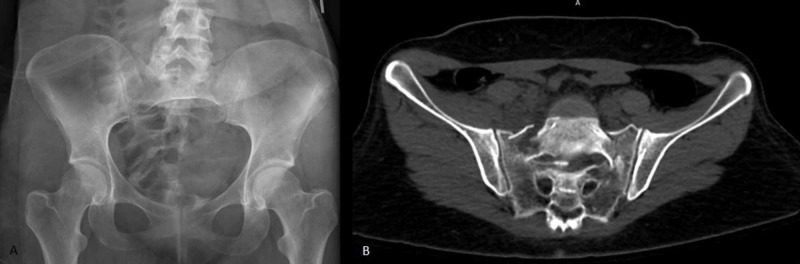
U-type sacral fracture injury films. (A) An AP pelvic radiograph of a U-type sacral fracture. (B) An axial view through the sacrum of a U-type sacral fracture
